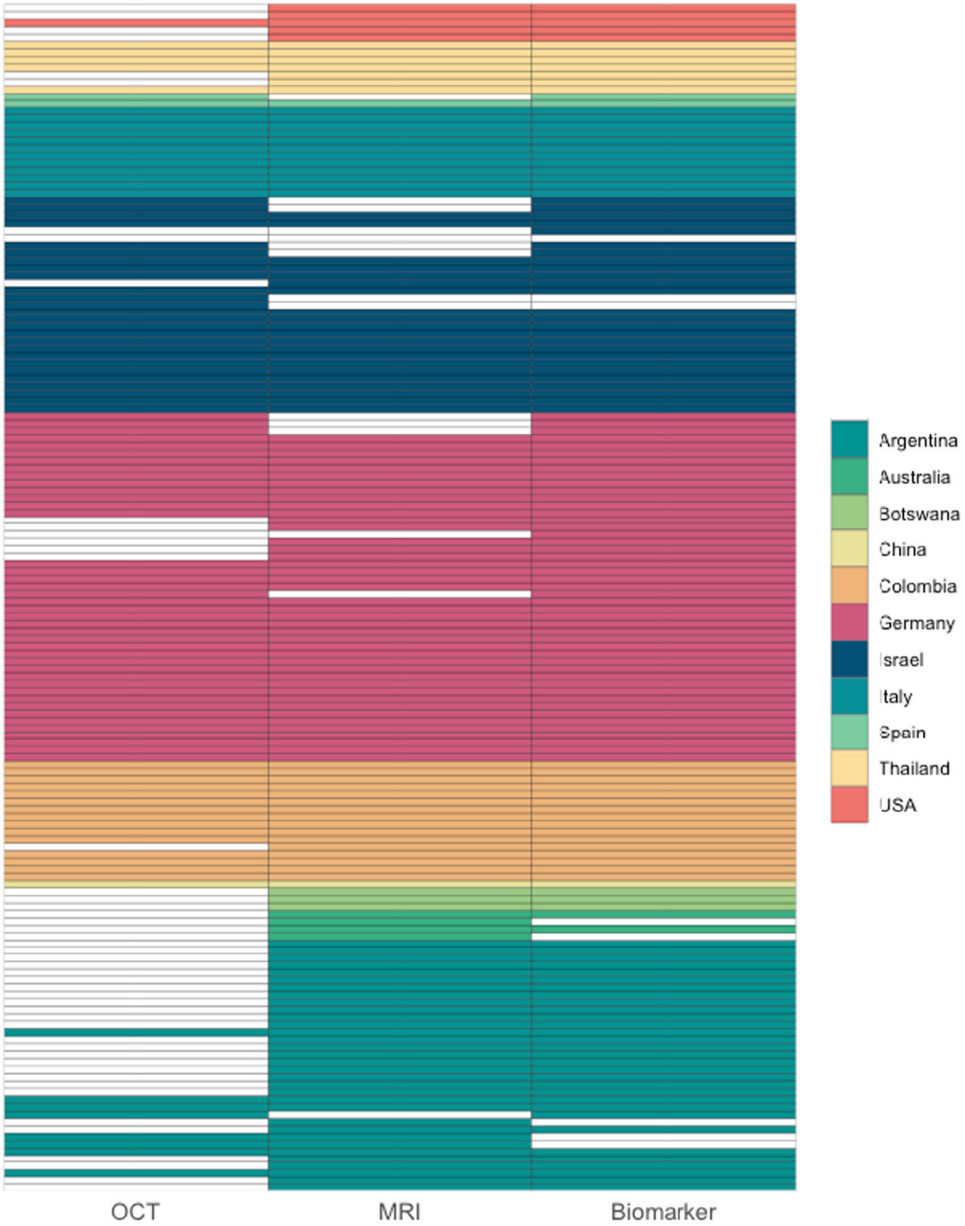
Availability of OCT, MRI, and biomarker (AQP4‐/MOG‐IgG, OCB) testing for all patients. Each row represents an individual patient. If any diagnostic procedure is not available, the respective tile is left blank. OCT was the least available paraclinical test in our cohort. AQP4‐IgG, aquaporin‐4‐IgG; MOG‐IgG, myelin oligodendrocyte glycoprotein‐IgG; MRI, magnetic resonance imaging; OCB, oligoclonal bands; OCT, optical coherence tomography.
